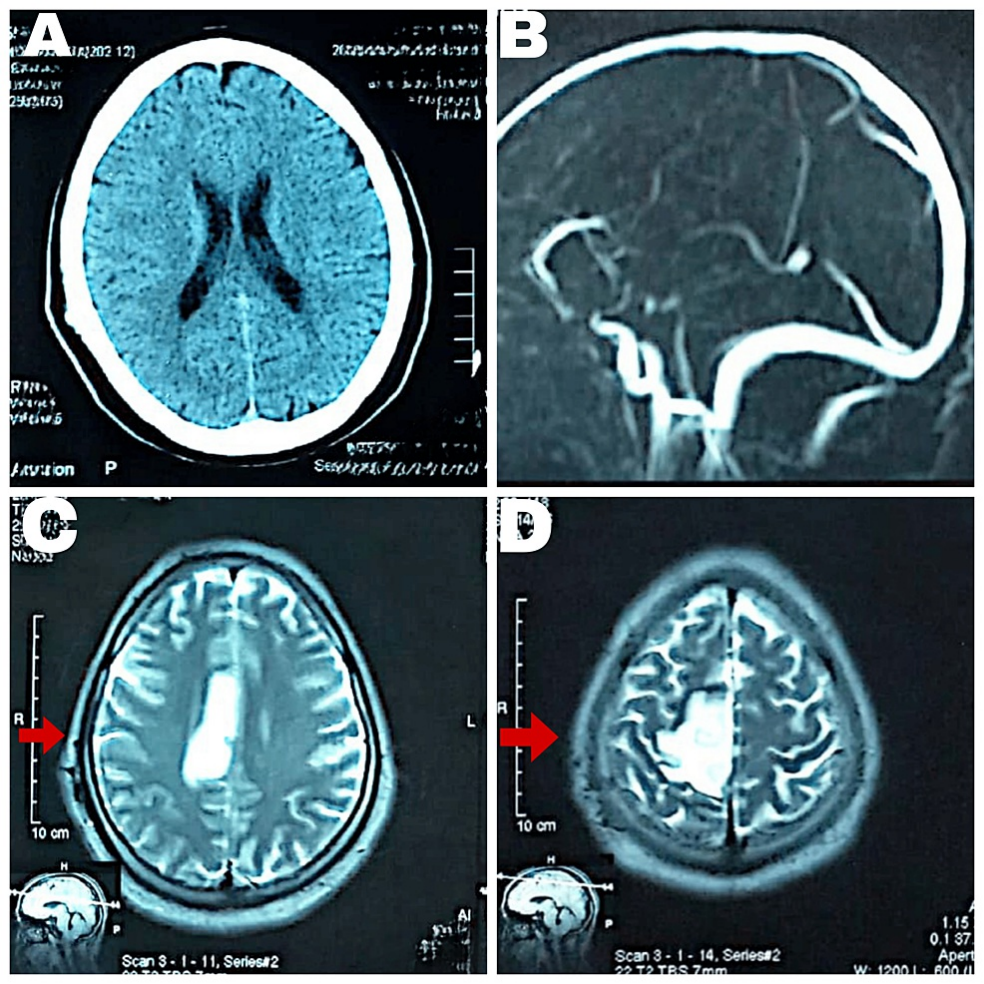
(A & B) Insignificant findings in the CT scan and magnetic resonance venography. (C & D) Ischemic infarct along the anterior cerebral artery in the MRI scan. The arrows show areas damaged by a vascular insult as a bright lesion in FLAIR imaging. FLAIR: fluid-attenuated inversion recovery
